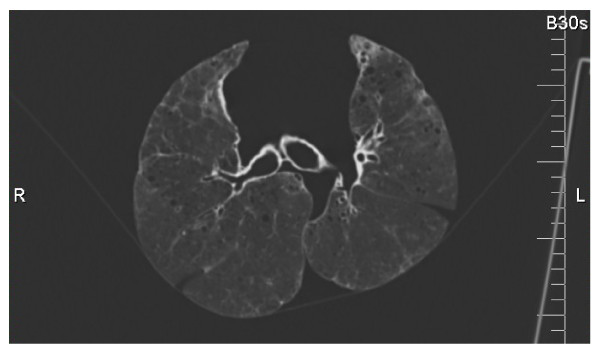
A sample CT image of an ex vivo human lung cradled in the specially designed hammock.
